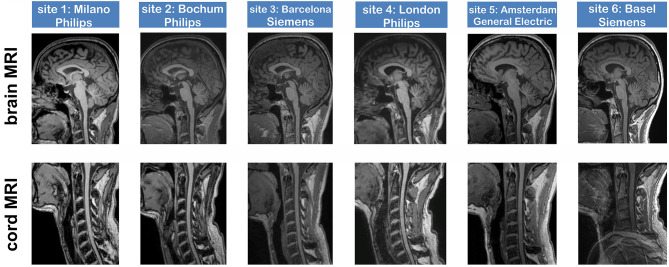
Image quality of 3D T1-weighted brain and spinal cord sequences at six 3-Tesla MRI scanners, acquired in the same healthy volunteer at all sites (age 45 years at first examination; male).
